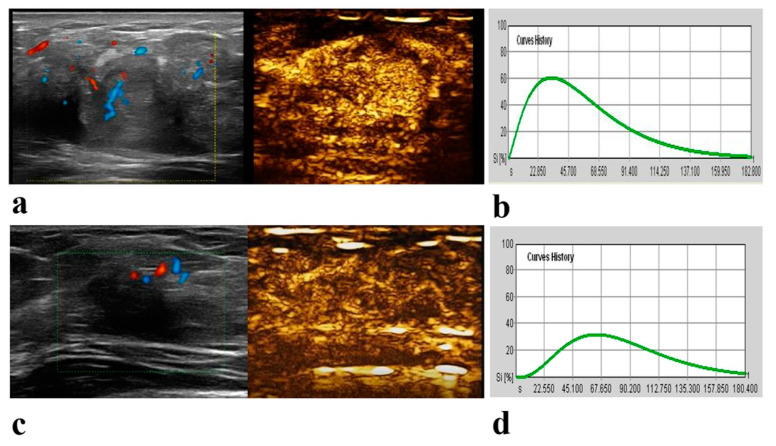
A 33-year-old woman with 4.0 cm triple-negative breast cancer of the right breast following neoadjuvant chemotherapy (NAC). Before NAC, Color Doppler US and contrast-enhanced ultrasound (CEUS) images obtained 38 s after contrast agent injection show an inhomogeneous hyper-enhancement lesion with an indistinct margin (a). The time-intensity curve (TIC) shows peak intensity (PEAK) is 61.70; time to peak (TTP), 32.26 s; regional blood volume (RBV), 4834.48; regional blood flow (RBF), 81.67, and mean transit time (MTT), 59.20 s. (b). After two cycles of NAC, Color Doppler US and CEUS images obtained 59 s after contrast agent injection show an inhomogeneous hyper-enhancement lesion with an indistinct margin (c). The TIC shows PEAK is 32.60; TTP, 63.19 s; RBV, 3271.80; RBF, 38.22, and MTT, 85.61 s (d). After surgery, pathological analysis found no residual cancer in the breast and sampled axillary lymph node. During 85 months of follow-up, there was no evidence of recurrence.
